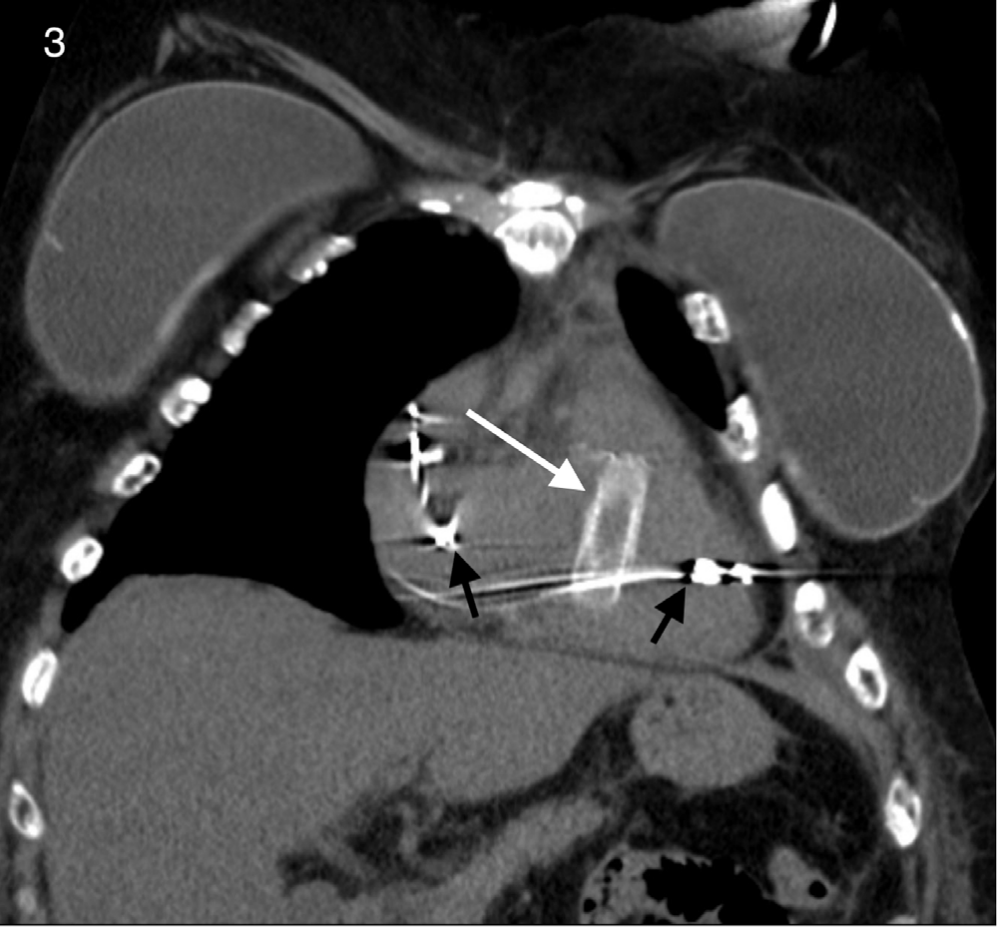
Coronal view CT demonstrating a migrated stent in the right ventricular outflow tract (white arrow). Black arrows indicate pacemaker leads placed for complete heart block.
